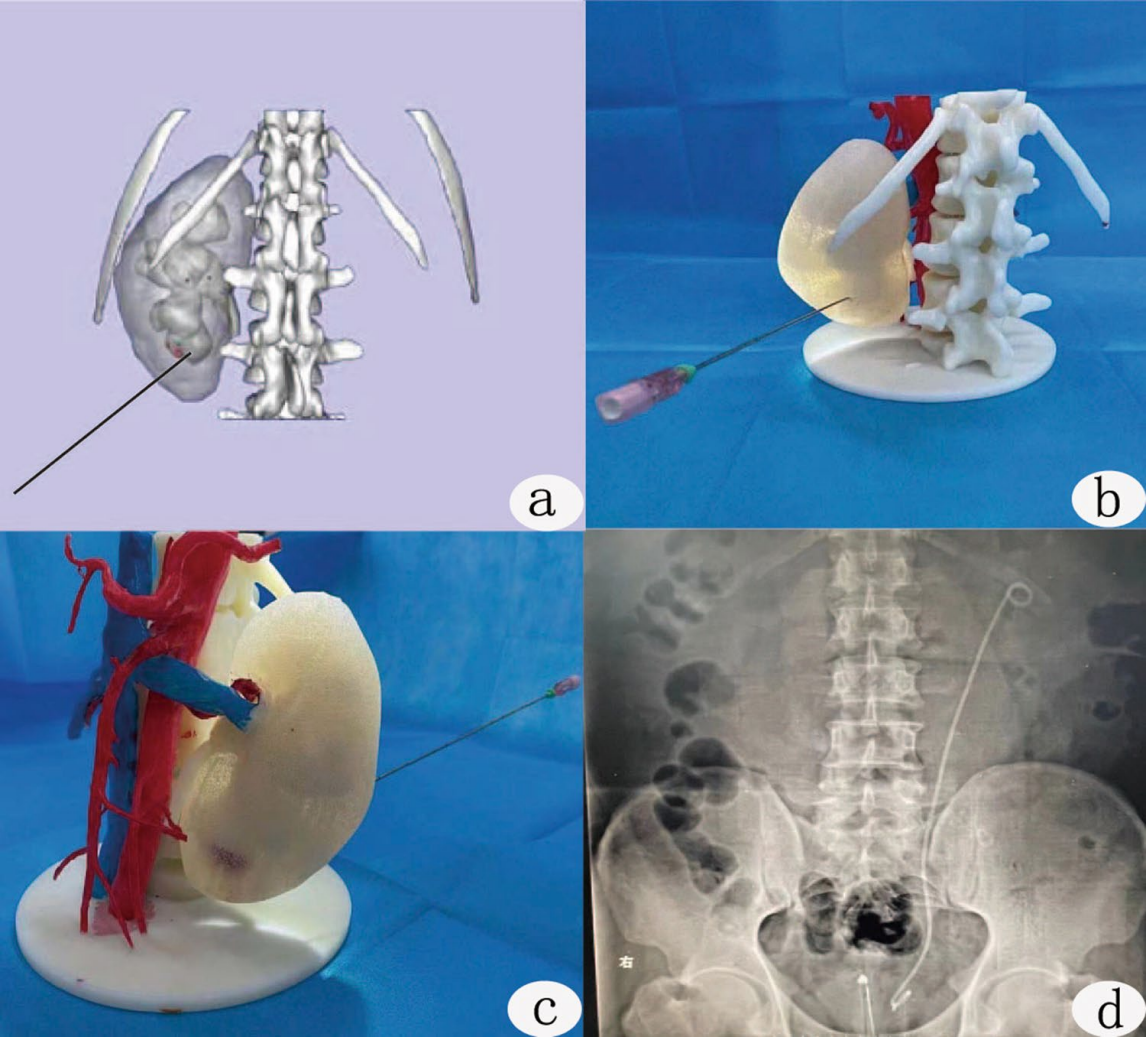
Puncture training and postoperative plain film. (a) Selection of puncture sites and calyces in a three-dimensional reconstruction image; (b,c) puncture training, target calyces, selection of puncture angle and estimation of puncture depth on 3D printed physical model. (d) Postoperative plain film.
